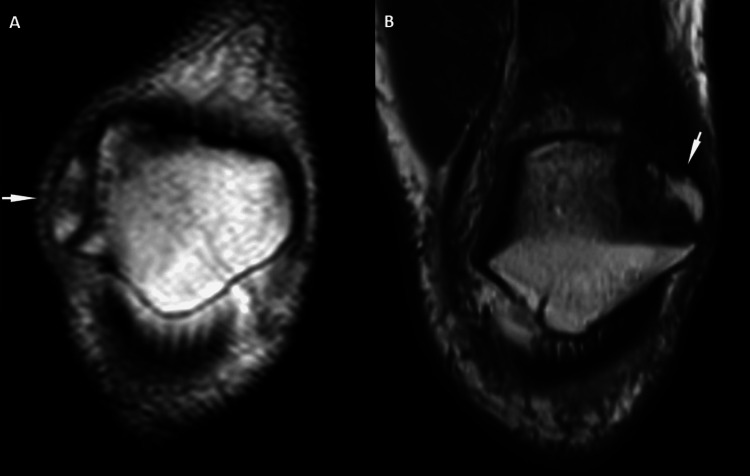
Bipartite Patella Types Coronal T1-weighted images show type II (A) and type III (B) bipartite patella (arrows).
